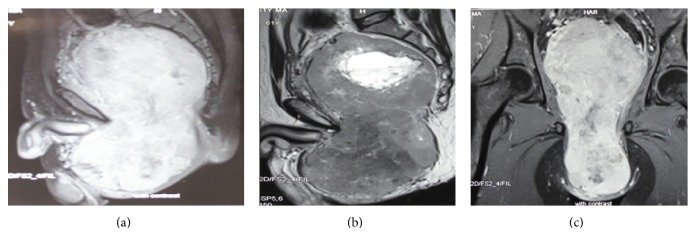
(a) T1W MRI sagittal section, (b) T2W MRI sagittal section, and (c) T1W MRI coronal section.
